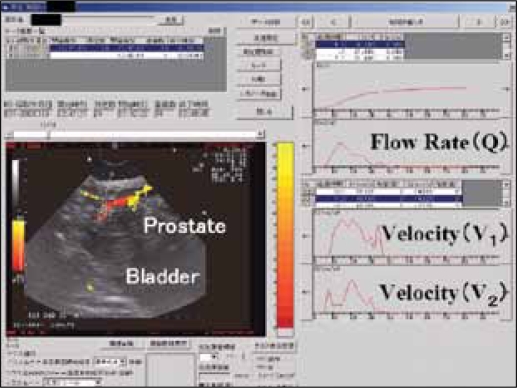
Voiding analysis of a case with benign prostatic enlargement: Left lower portion: A retrieved picture obtained from Doppler ultrasound, which demonstrated an enlarged prostatic nodule. Note that although the distal prostatic urethra was clearly identified from the prostatic nodule, the proximal site of the prostatic urethra did not show a Doppler signal. Right lower portion: Flow velocity curve obtained by Doppler ultrasound. Right upper portion: Flow rate curve obtained by uroflowmetry.
